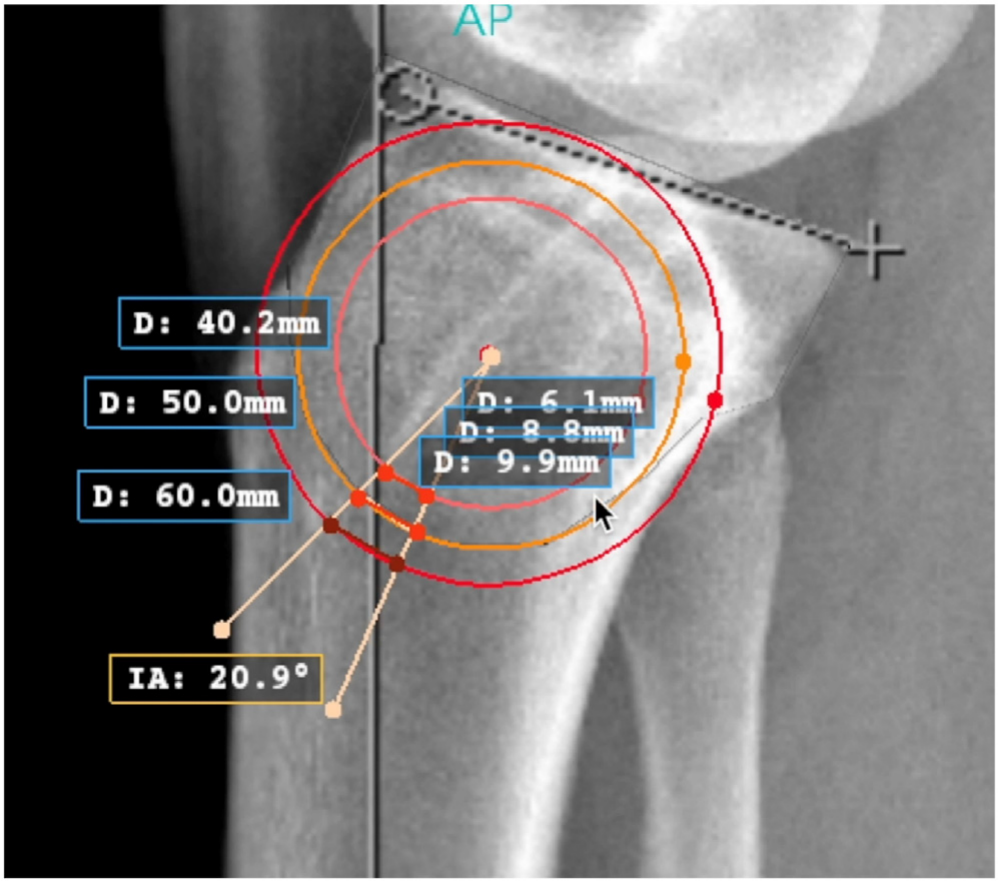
On a lateral radiograph of the right knee the desired correction (IA: 20.9°) is labeled. To measure the amount of rotational correction needed, measure the distance between the points at which each angle arm subtends a circle that represents a proposed osteotomy curve. If the red circle (D: 60 mm) is the template used to create the osteotomy, a rotational correction of (D: 9.9 mm) is needed to correct the slope (IA: 20.9°). Similarly, if the orange circle (D: 50 mm) represents the osteotomy, a rotational correction of (D: 8.8 mm) is needed. If the pink circle (D: 40 mm) represents the osteotomy, a rotational correction of (D: 6.1 mm) is needed. (D, diameter; IA, posterior tibial slope measure.)
